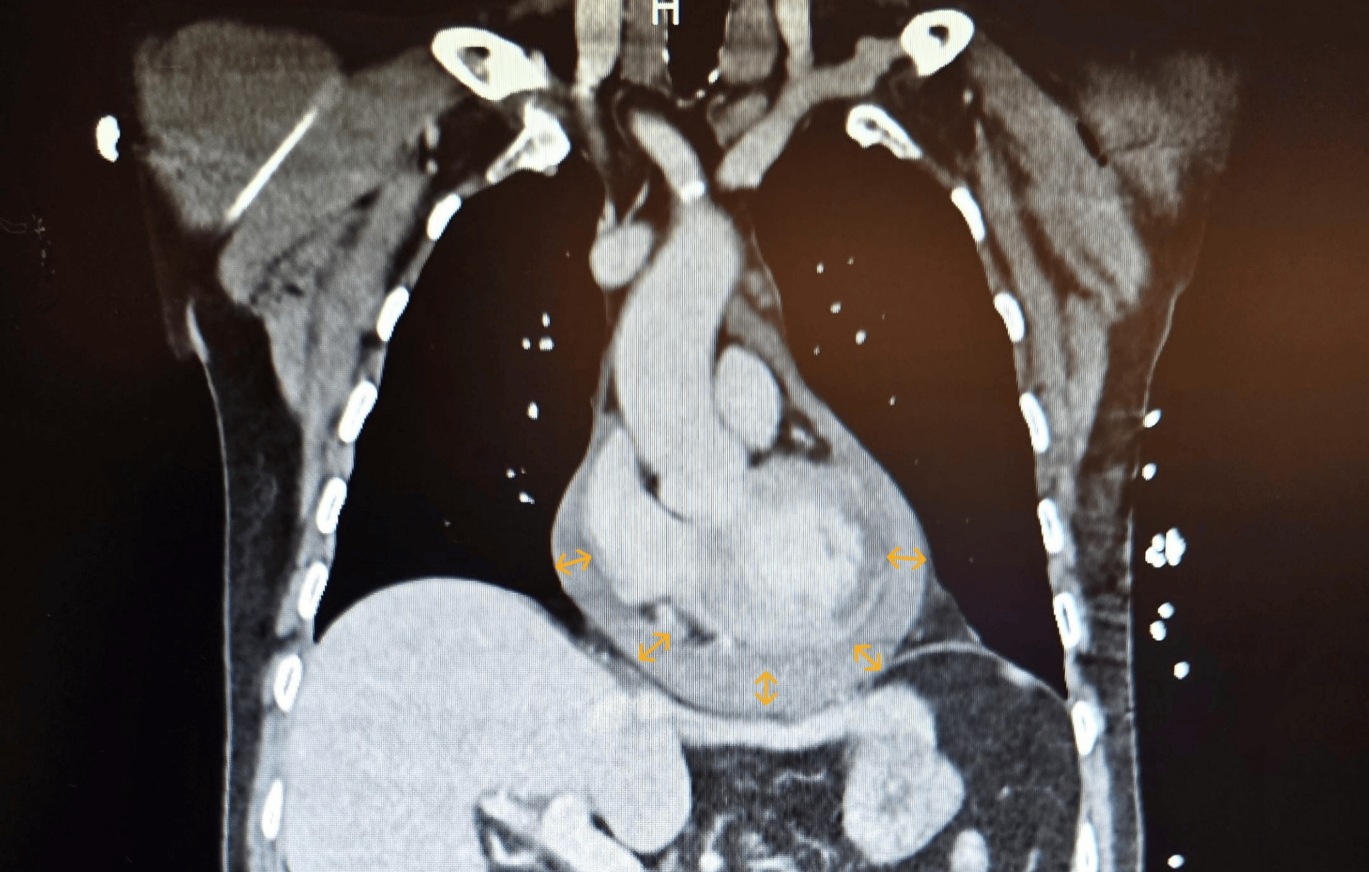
Frontal section of chest CT scan. Yellow arrows show pericardial effusion.
